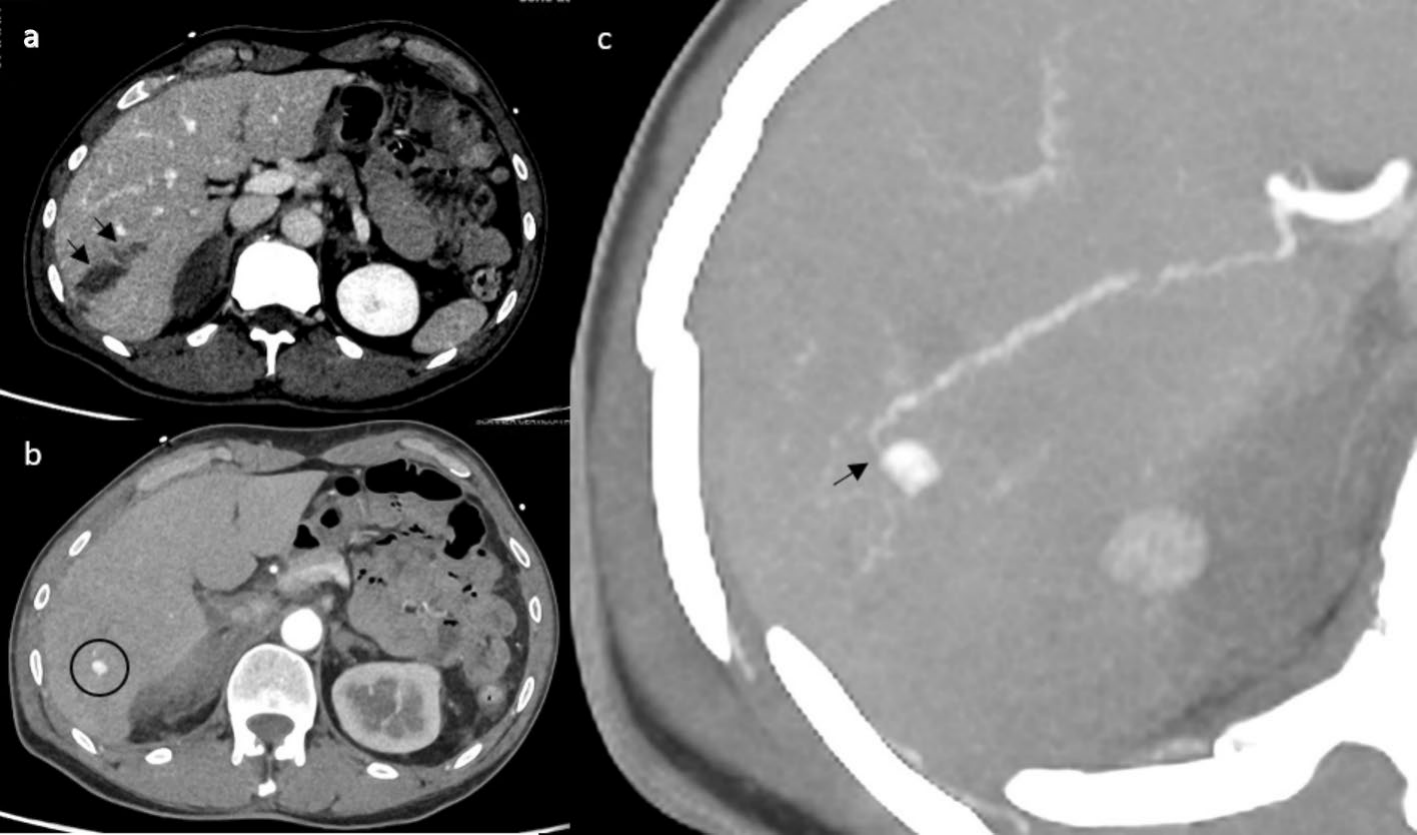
a Axial CT tri-phasic of the abdomen shows an irregular linear/branching area of hypoattenuation representing laceration (black arrows) in the hepatic segment VI in portal phase. b A query hyper-enhancing lesion measuring about 7 mm is seen in segment VI suspected to be a pseudoaneurysm in arterial phase (black circle). c Axial CT Angiography of the abdomen with maximum intensity projection (MIP) confirms the previously noted hyper enhancing lesion with close relation with a branch from the right hepatic artery (black arrow)
